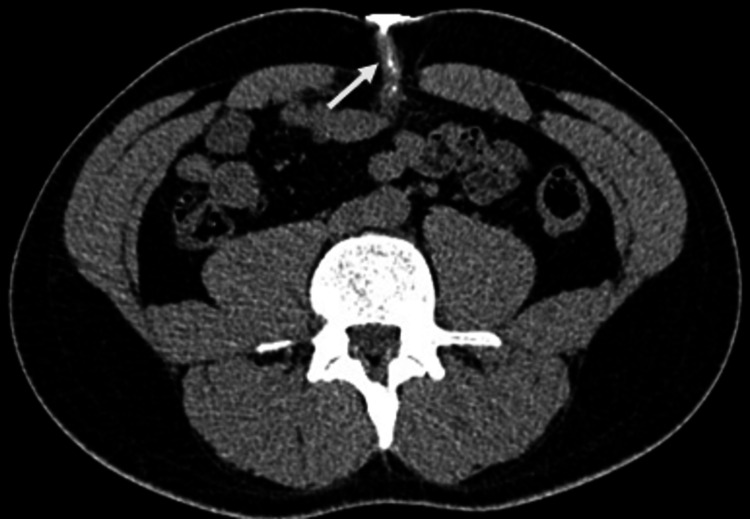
Axial abdominal computed tomography image following iodinated contrast instillation through umbilical opening shows a blind ending partially opacified tubular collection (White arrow) extending posteriorly from the umbilicus towards bladder dome.
